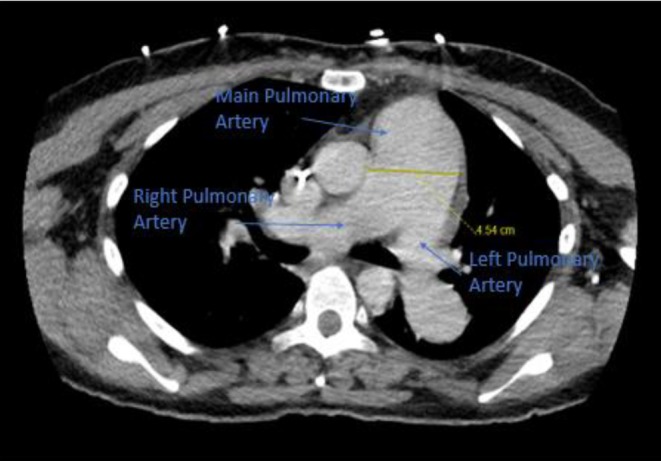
CT chest with contrast showing enlarged pulmonary artery measuring 4.5 cm.
